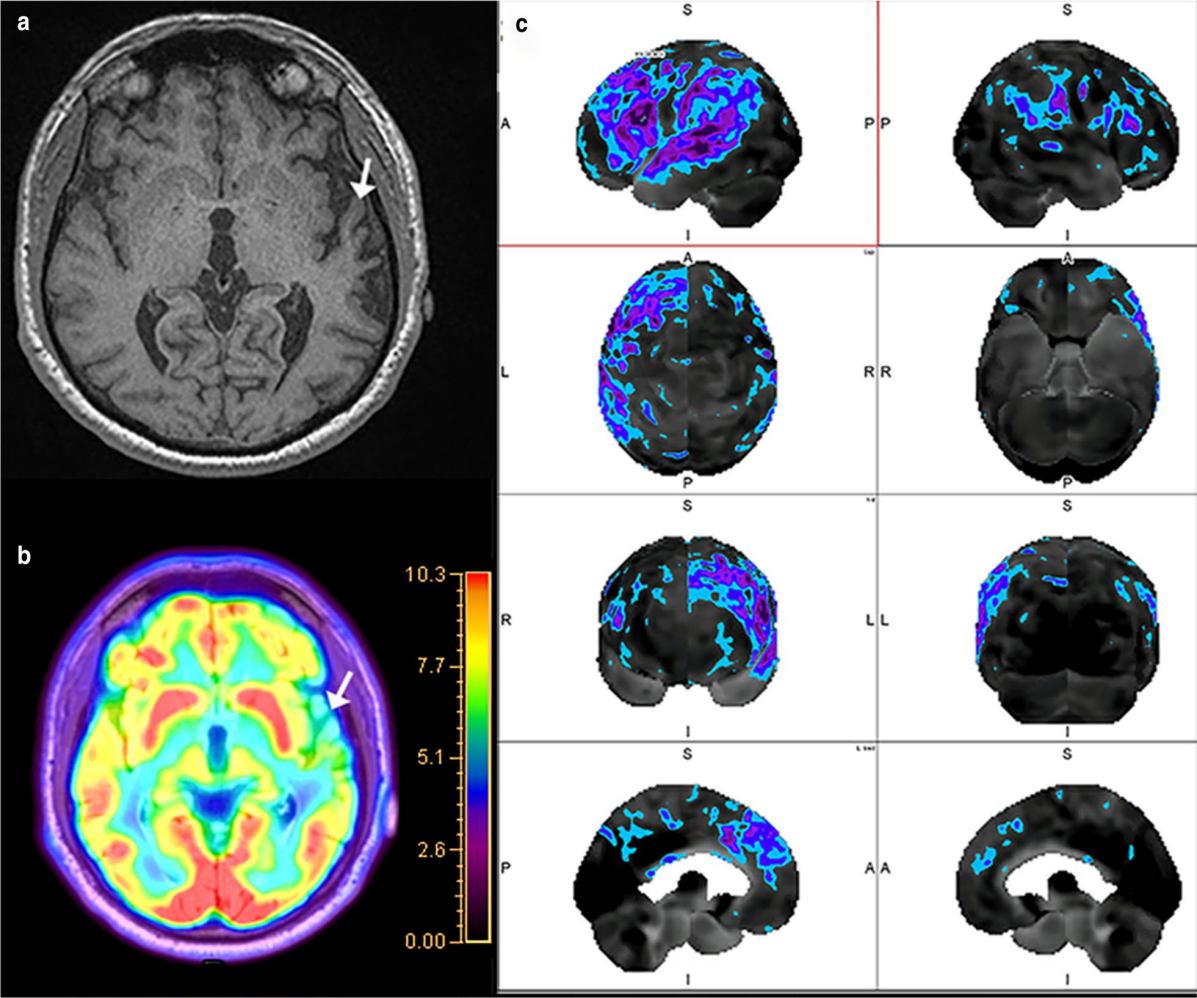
A 58-year-old man with notable agrammatism, impaired comprehension and repetition of syntactically complex sentences, and spared single word comprehension, Montreal Cognitive Assessment (MoCA) 16/30. T1-weighted volumetric image (a) demonstrates asymmetric atrophy of the left perisylvian and insular cortices (arrow). Fluorodeoxyglucose PET/MRI demonstrates hypometabolism in this region (arrow in b). Single-subject statistical parametric mapping of the FDG-PET/MRI (c) demonstrates the asymmetric left greater than right hypometabolism involving the frontal and temporal operculum and parietal lobes with sparing of the PCC and precuneus (as before, areas of blue progressing to purple represent further negative deviation from the mean uptake values of controls). Combining the clinical and imaging data, a diagnosis of nfPPA was rendered
